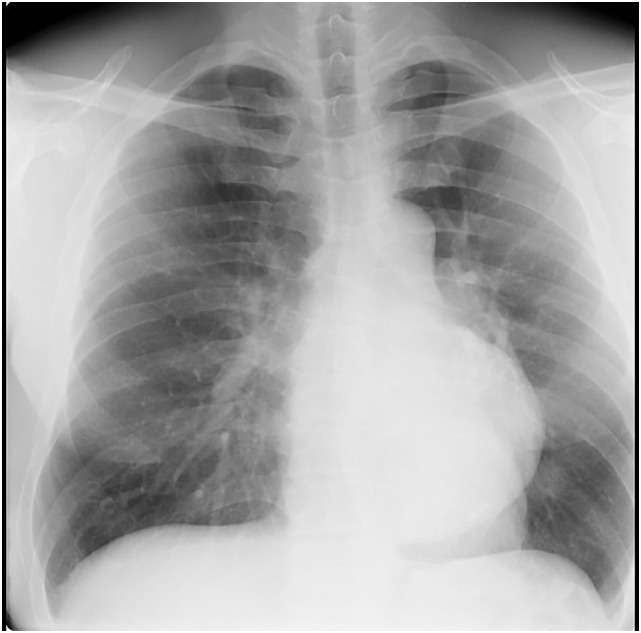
Chest X-ray findings of the patient. Chest X-ray film showed a mass in the left hilar region extending toward the lower mediastinum.
